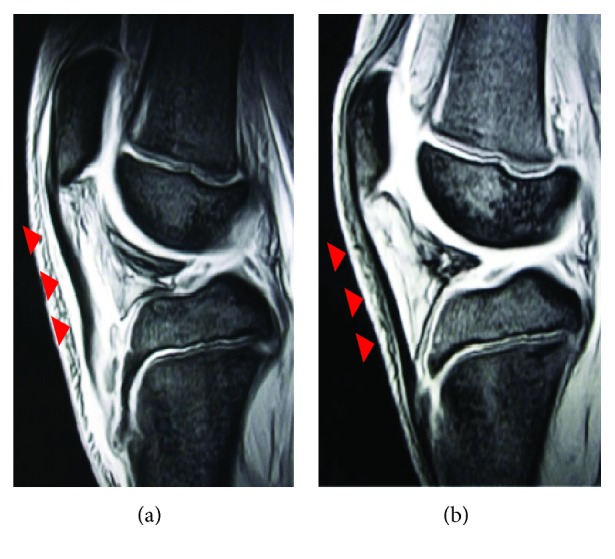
(a) Left is affected side. (b) Right is healthy side. Magnetic resonance imaging (MRI) revealed swelling and loosening of the patellar tendon.
